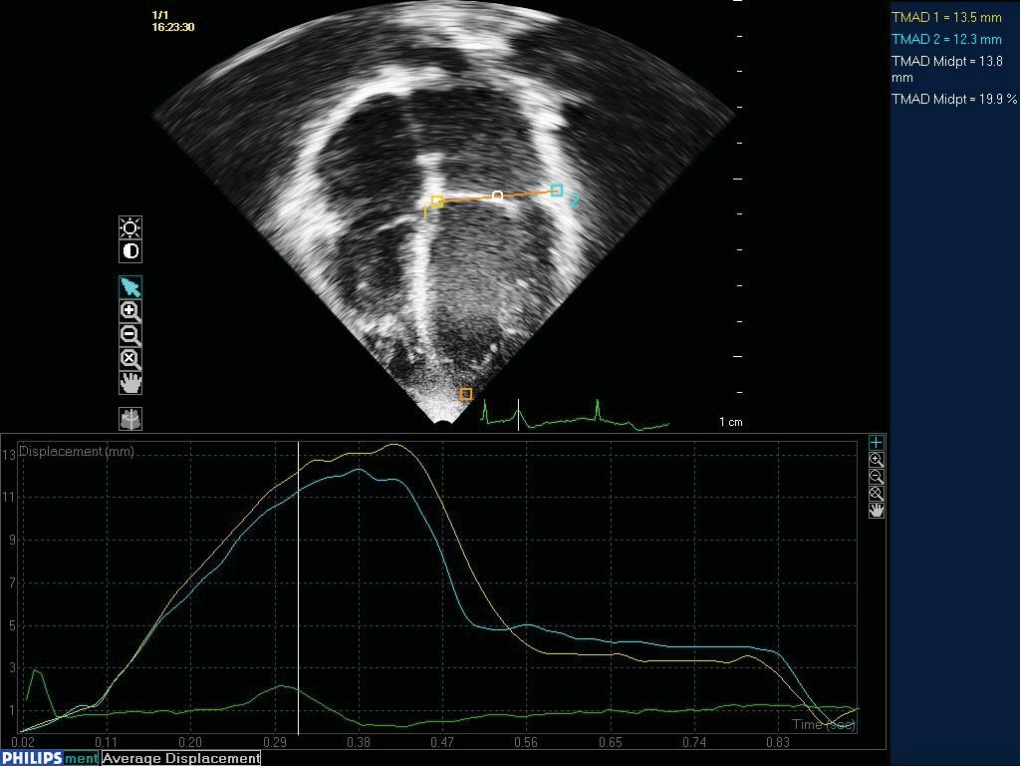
Four chamber apical view using two dimensional trans-thoracic echocardiography. Three points are manually selected at the medial
